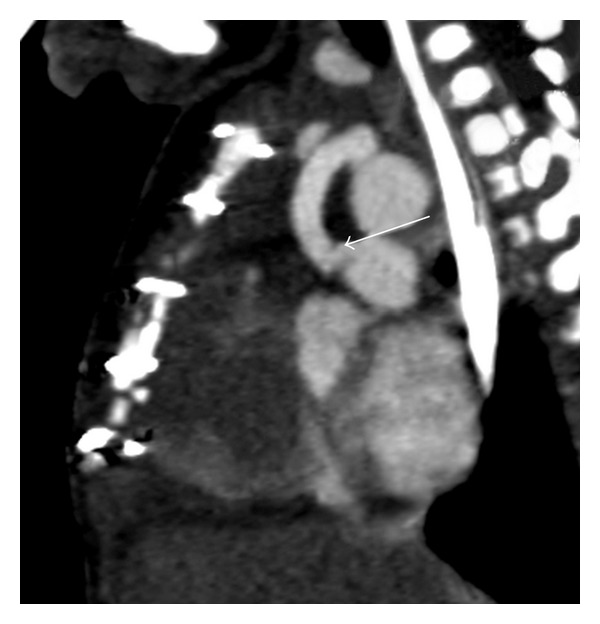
CT angiography (sagittal view) demonstrating a narrowing of the modified BT shunt at the point of anastamosis with the pulmonary artery.
